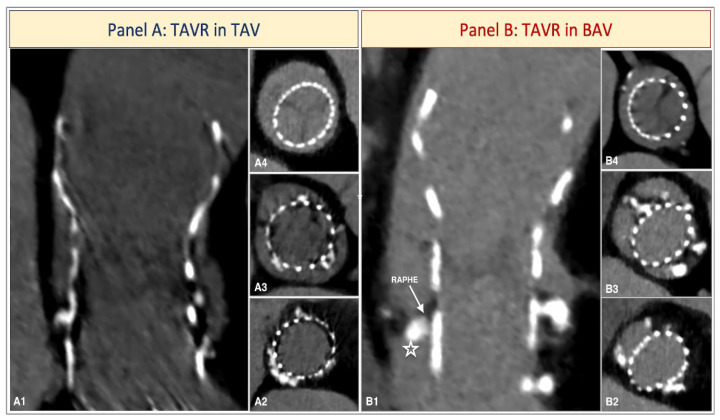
Comparison of prosthesis anchoring in tricuspid aortic valve (TAV) and bicuspid aortic valve (BAV). (Panel A): Post-TAVR CT scan of a 34 mm CoreValve Evolut R in a patient with tricuspid aortic valve. The long-axis view (A1) shows adequate expansion of the prosthesis, which appears circular in all planes displayed in the short-axis views: at the VBR (A2), where anchoring occurs through a 360-degree interaction between the prosthesis and the aortic annulus; at sinus plane (A3); at coaptation leaflet plane (A4). (Panel B): Post-TAVR CT scan of a 26 mm Corevalve Evolut R in patient with a bicuspid aortic valve. The long-axis view (B1) reveals that the prosthesis does not fully interact with the LVOT (white star) but instead anchors at the raphe. This observation is confirmed in the short-axis views, where the prosthesis only partially adheres to the VBR (B2), while it anchors at the level of the raphe and is moved towards the opposite commissure (B3). In both B2,B3 short-axis views, the prosthesis has an elliptical shape; however, at the coaptation leaflet plane (B4), it gains a proper circularity, not hampering prosthesis’ performance.
